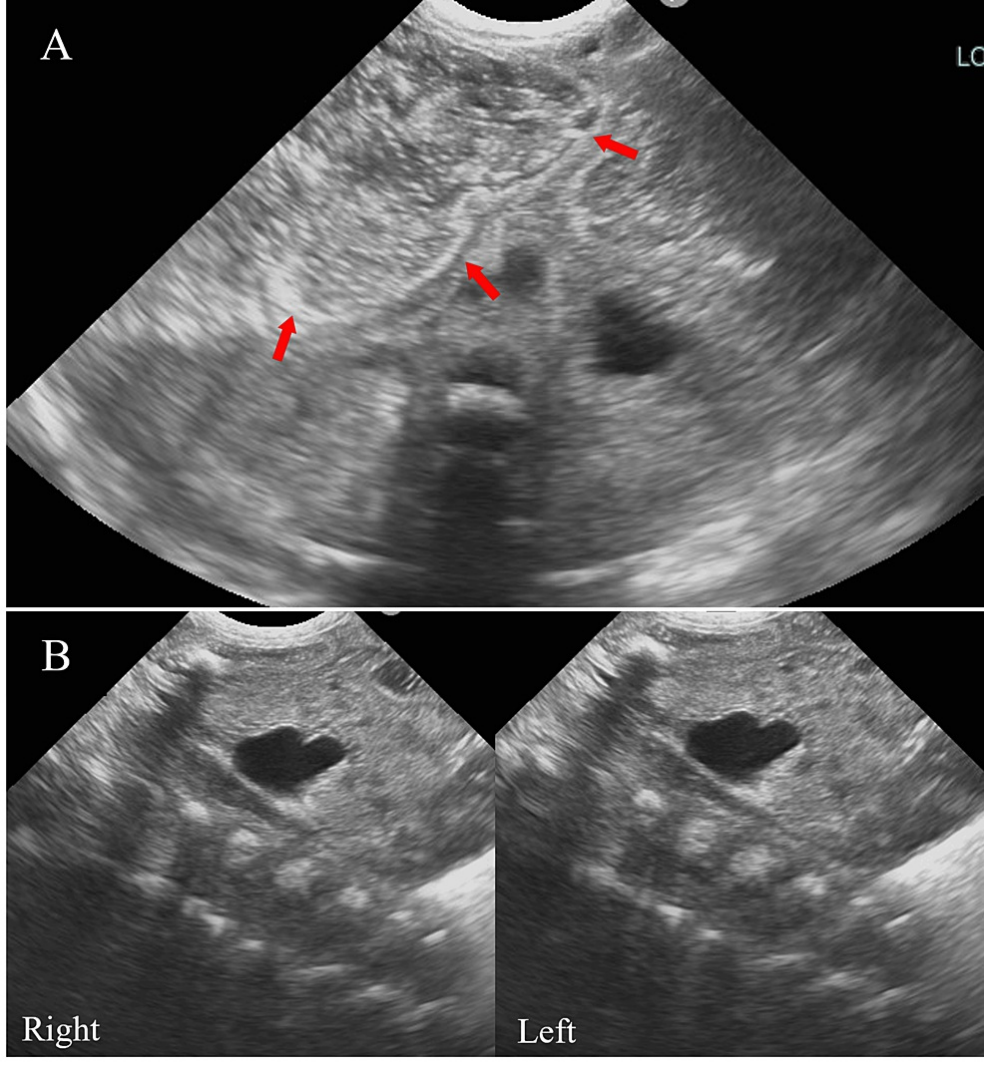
Ultrasonography of the abdomen (A) Image showing numerous small hyperintense nodules with acoustic shadows in the dilated intestinal tract (arrows). (B) Despite the presence of mild bilateral hydronephrosis, the longitudinal diameter of the kidneys is normal.
